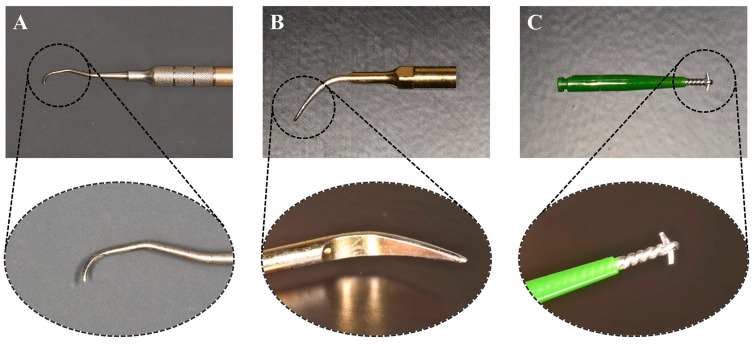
Medical devices used for mechanical debridement treatment. (A) Gracey curette. (B) Ultrasonic Ti scaler. (C) Titanium brush.
